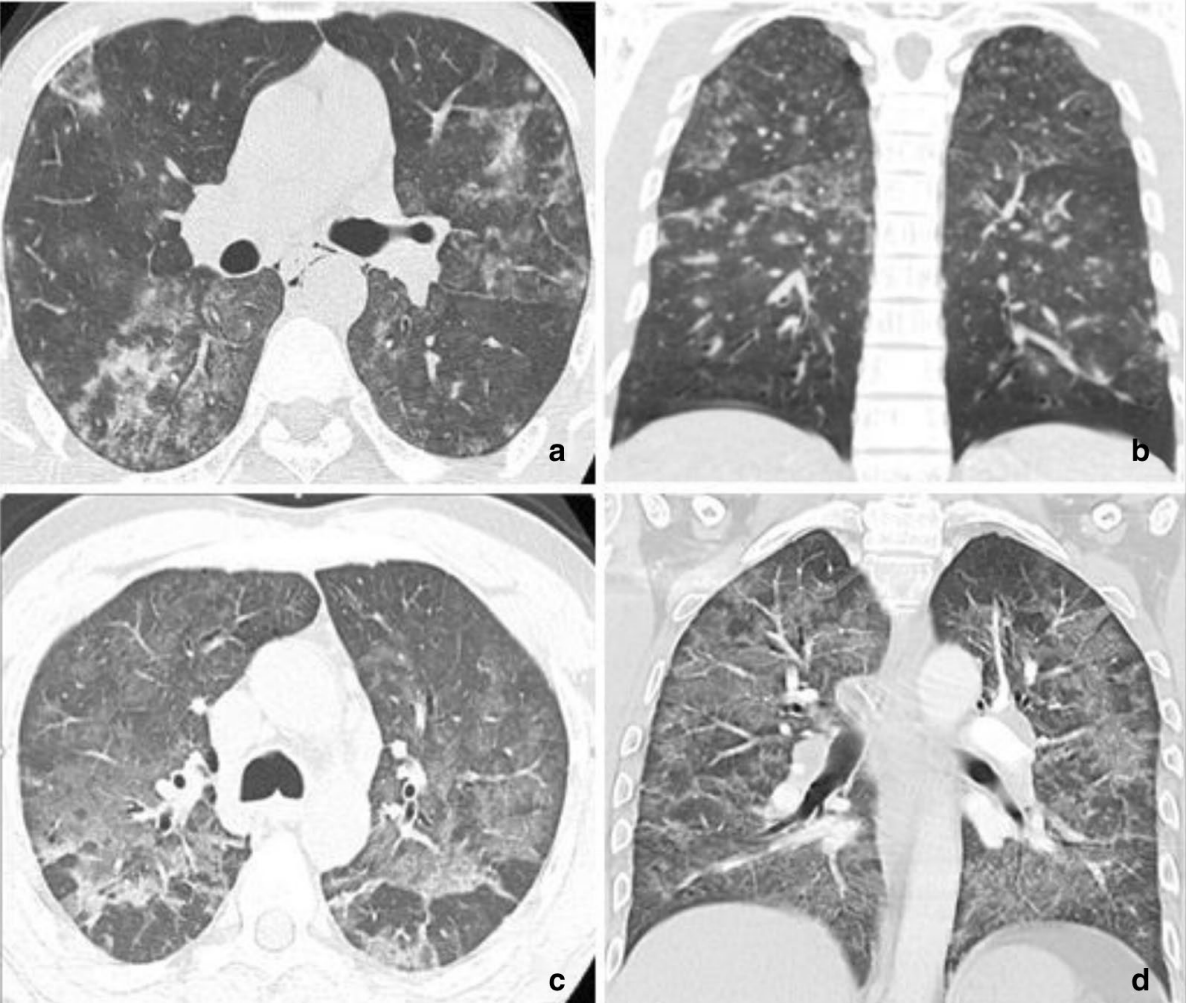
Non-infectious mimickers of indeterminate COVID-19. a, b A 33-year-old bird breeder with subacute hypersensitivity pneumonitis, axial and coronal reformatted CT images shows patchy or diffuse bilateral ground-glass opacities associated with poorly defined centrilobular nodules and lobular areas of reduced attenuation indicative of air trapping. c, d A 38-year-old man with acute eosinophilic pneumonia, axial and coronal reformatted CT images shows multifocal non-rounded GOO associated with inter- and intralobular septal thickening and thickened bronchovascular bundles
